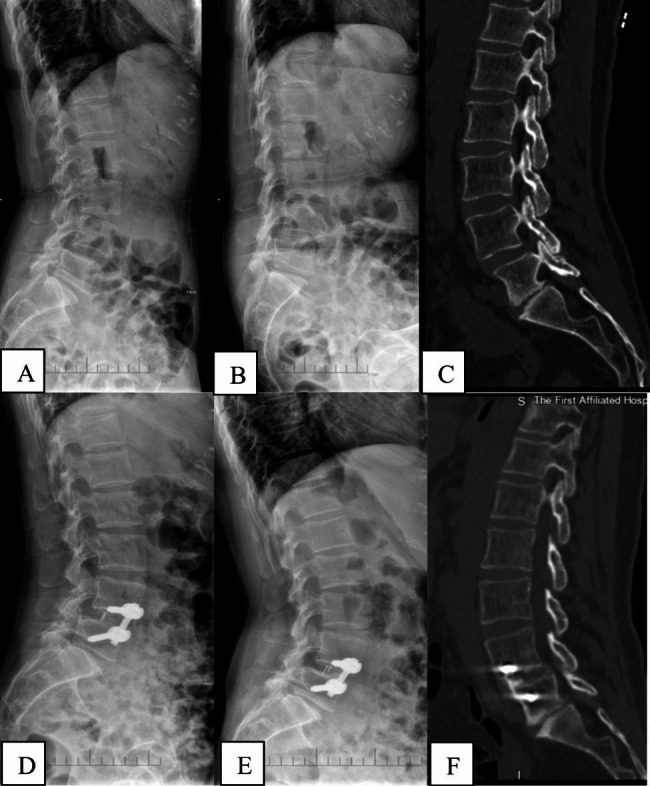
Images of a 59-year old female patient who was diagnosed with osteopenia. (A)-(C).Preoperative lateral radiograph and CT show the L4/5 degenerative spondylolisthesis. (D)-(F). Postoperative lateral radiograph and CT showed the surgery provided reduction and fixation with satisfactory clinical results and relatively high fusion rate. (D:Postop 3d; E、F: follow-up at 1 year postoperatively)
